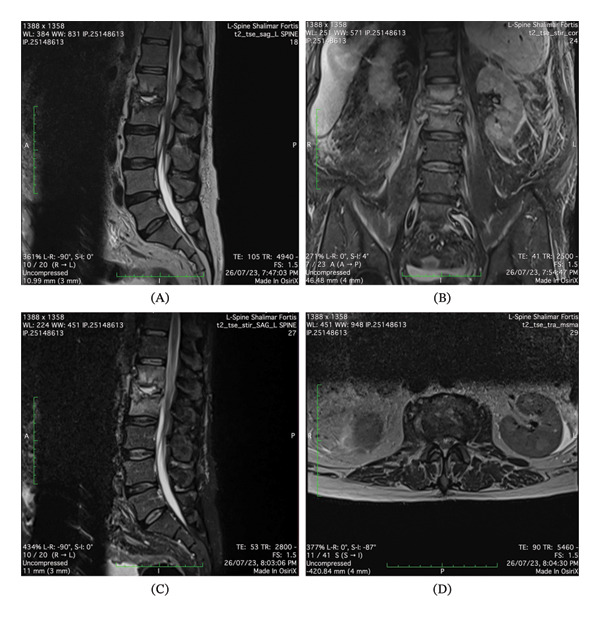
MRI images obtained after the onset of neurological deficit. (A) T2 sagittal and (B) T2 coronal images demonstrating increased signal hyperintensity along the end plates and increased cord compression relative to initial imaging. (C) Sagittal and (D) axial T1 contrast images showing increased contrast enhancement with end plate destruction and progressive neural compression.
